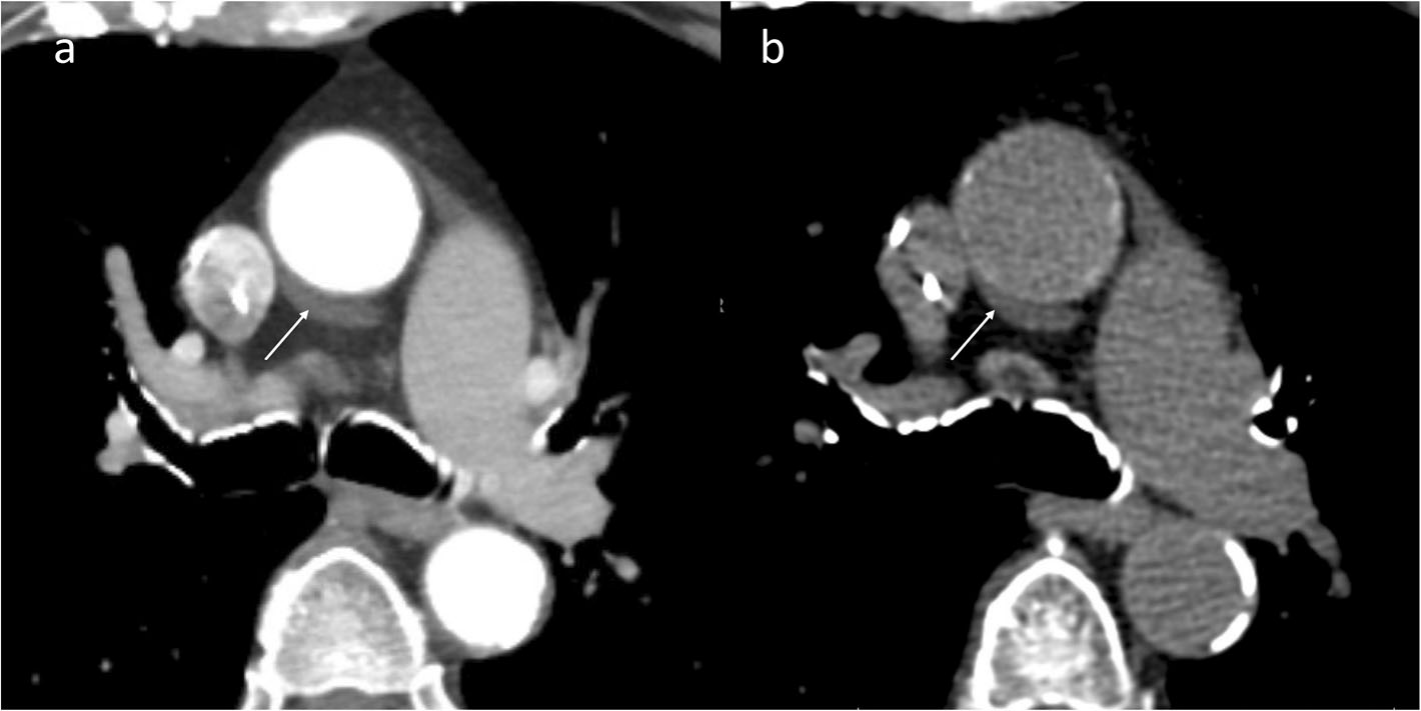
A 65-year-old male with acute chest pain. Contrast enhanced axial CTA (a) image shows asymmetrical hypodensity adjacent to right lateral wall of ascending aorta (arrow). Non-contrast CT (b) confirmed hypodense fluid attenuation consistent with superior pericardial recess; potential mimic of intramural hematoma
